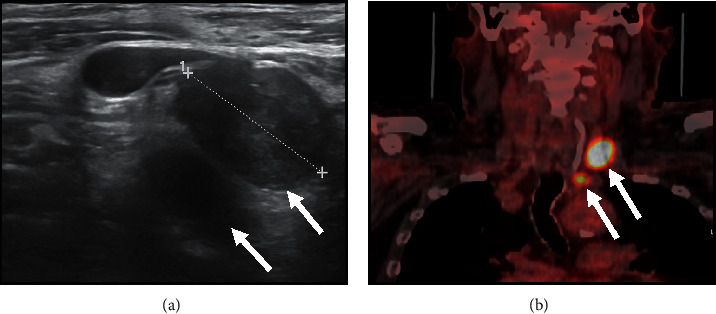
Ultrasonographic features of second nodal recurrences (6 years after surgery and radioactive iodine 131I therapy) of papillary thyroid carcinoma (two laterocervical metastatic hypoechoic lymph nodes, 2.2 cm and 1 cm, respectively) with irregular shape, no visible hilum and focal hyperechogenicity ((a), arrows). At 18F-FDG PET/CT, both lymph nodes showed significant tracer uptake (arrows) (the larger one: SUV max: 20.7; the smaller one: SUV max: 11) (b).
